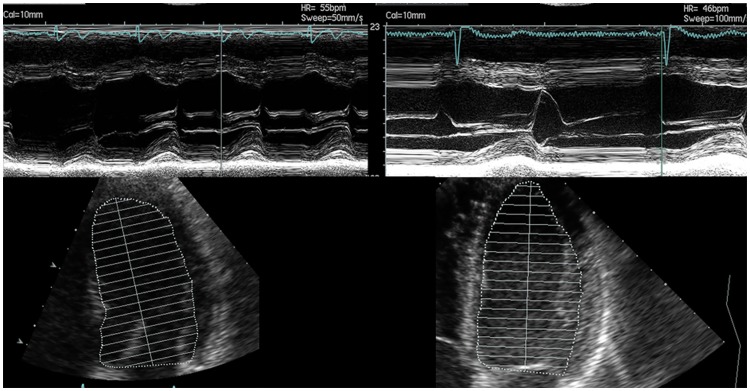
Echocardiography images of M-mode and apical two-chamber views. Athlete 1 was classified as eccentric LVH with 2TC due to a LVID of 57.1 mm and a PWT of 9.8 mm resulting in a relative wall thickness of 0.34. He was reclassified with 4TC to concentric non-dilated LVH due to a relative short LV length of 8.2 cm and an associated LV EDV/BSA of 60.1 ml/m^2^ resulting in a Concentricity of 10.4 ml/m^2/3^. Athlete 2 was also classified as eccentric LVH with 2TC due to a LVID of 53.6 mm and a PWT of 10.6 mm resulting in a relative wall thickness of 0.40. In contrast to Athlete 1, Athlete 2 was reclassified with 4TC to eccentric non-dilated LVH due to a longer LV length of 9.3 cm and an associated higher LV EDV/BSA of 71.5 ml/m^2^ resulting in a smaller Concentricity of 8.7 ml/m^2/3^.
